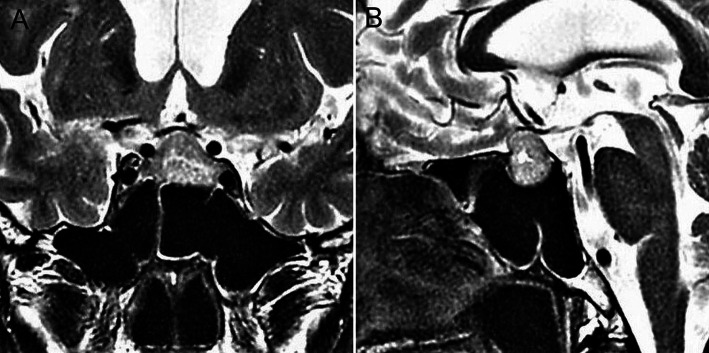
Preoperative coronal (A) and sagittal (B) T2-weighted magnetic resonance images showing a pituitary tumor as slight hyperintensity, compressing the optic nerve.
